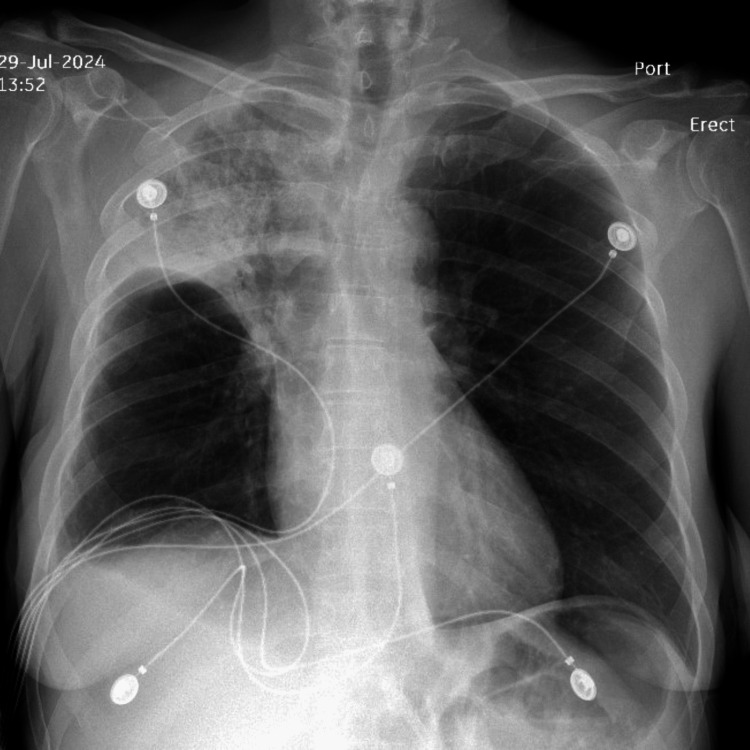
Chest radiograph obtained prior to discharge showing further resolution of right upper lobe consolidation.
